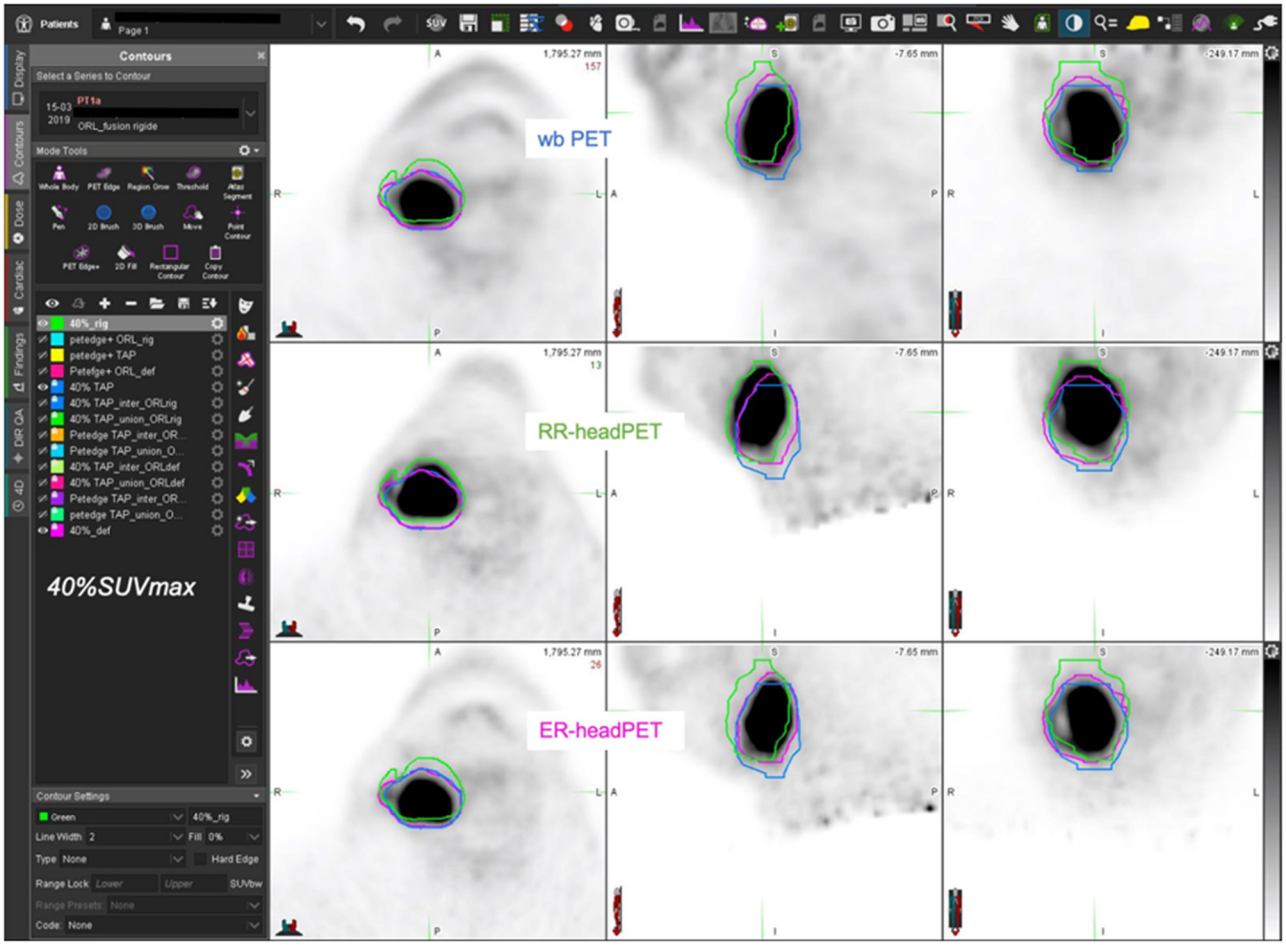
59-year-old man with T4 N1 M0 oropharyngeal SCC (AJCC stage IV) – estimated MTV = 49.0cc. wbPET MTV (=V1 in blue), RR-headPET MTV (=V2 in green) and ER-headPET MTV (=V3 in pink) delineated with 40%SUVmax segmentation method reported on the wbPET (top row), RR-headPET (middle row) and ER-headPET (bottom row) images in axial (left column), sagittal (center column) and coronal (right column) views. OF (V3, V1) = 86.6% vs. OF (V2, V1) = 59.4%; DICE (V3, V1) = 83.2% vs. DICE (V2, V1) = 60.5%.
